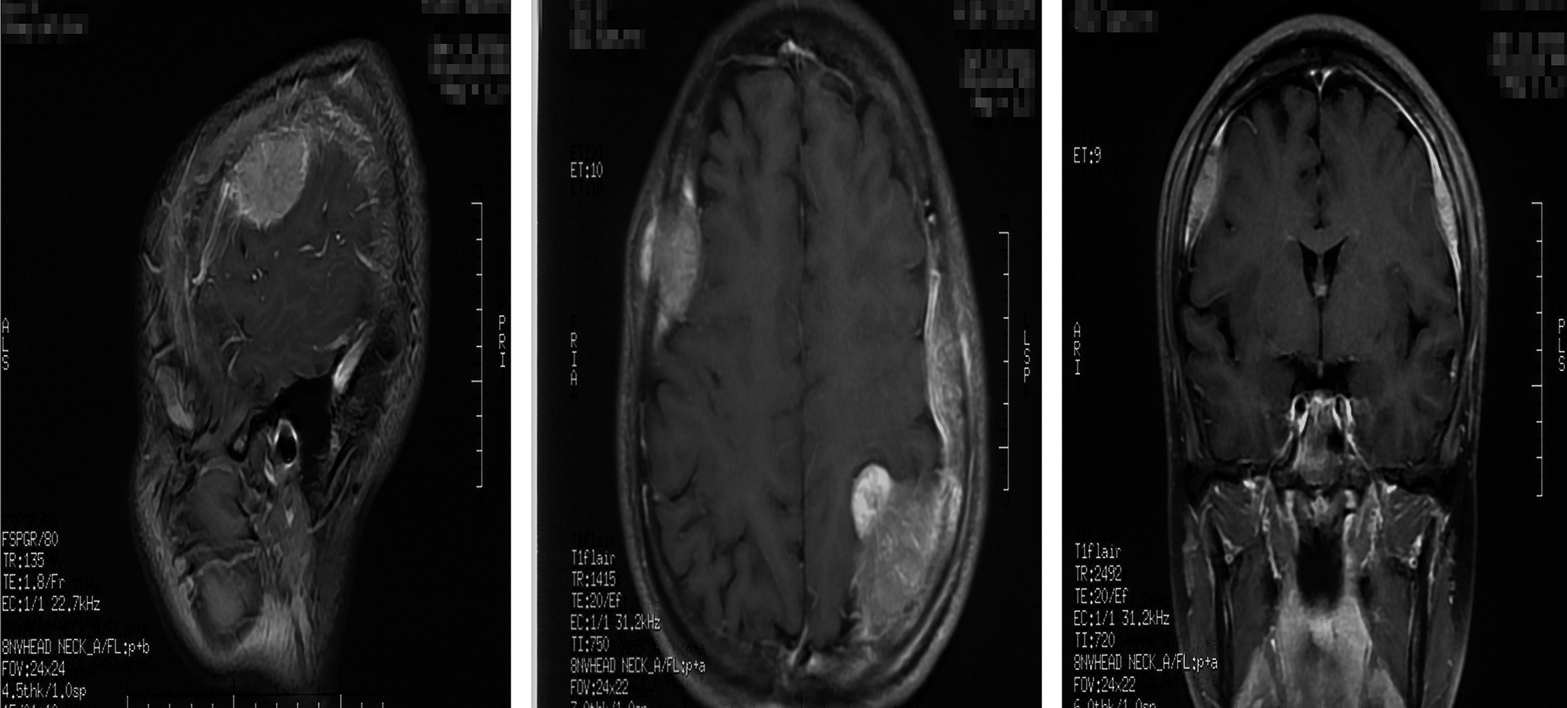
MRI of the brain showed the metastases of the scalp, skull, and meninges.
